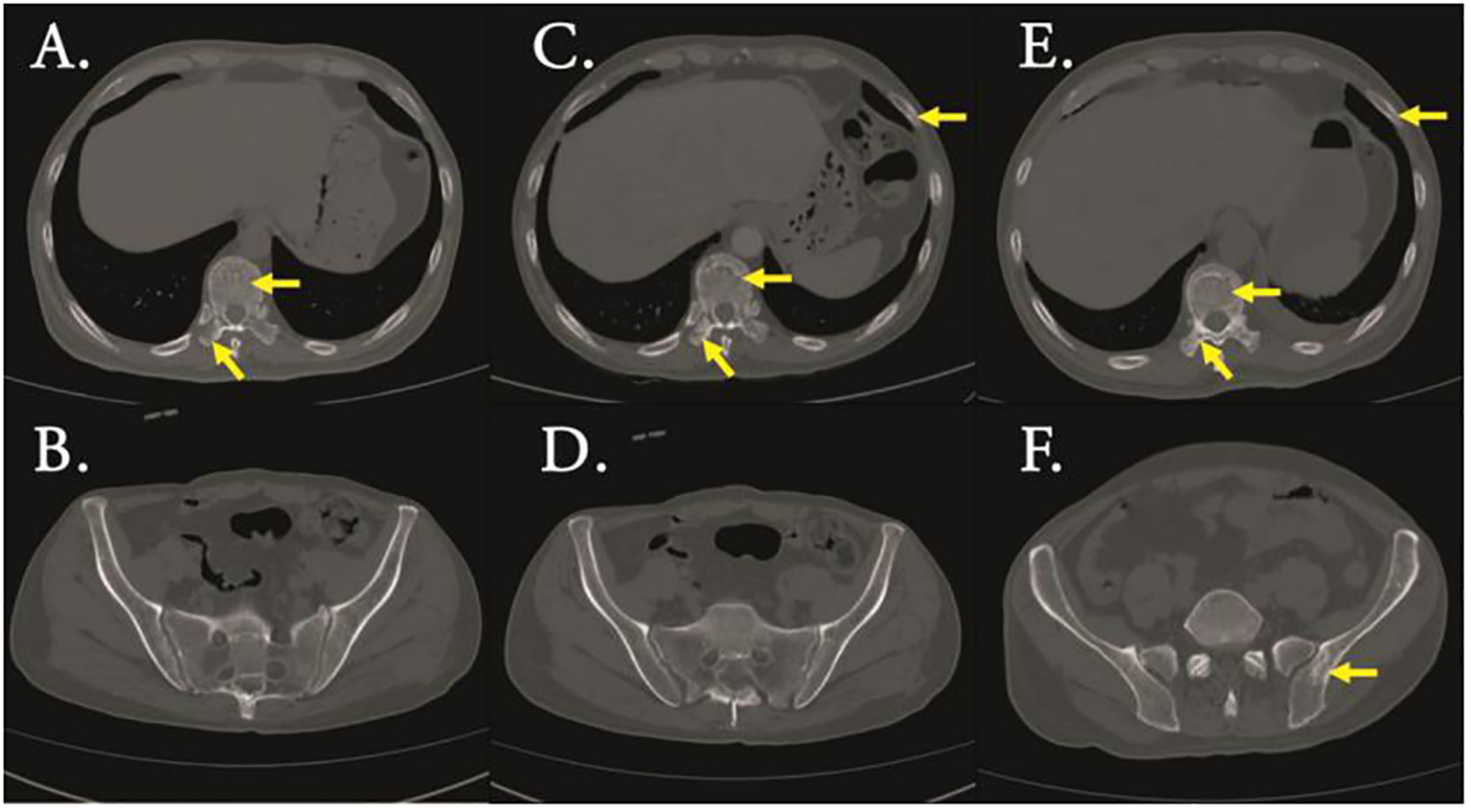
CT scan (bone window) revealed bone destruction of Thoracic vertebrae and transverse processes (A) and ilium (B) of initial diagnosis,bone destruction of Thoracic vertebrae, transverse processes and rib (C) and ilium (D) of complete remission(CR),bone destruction of Thoracic vertebrae, transverse processes and rib (E) and ilium (F) of relapse.
